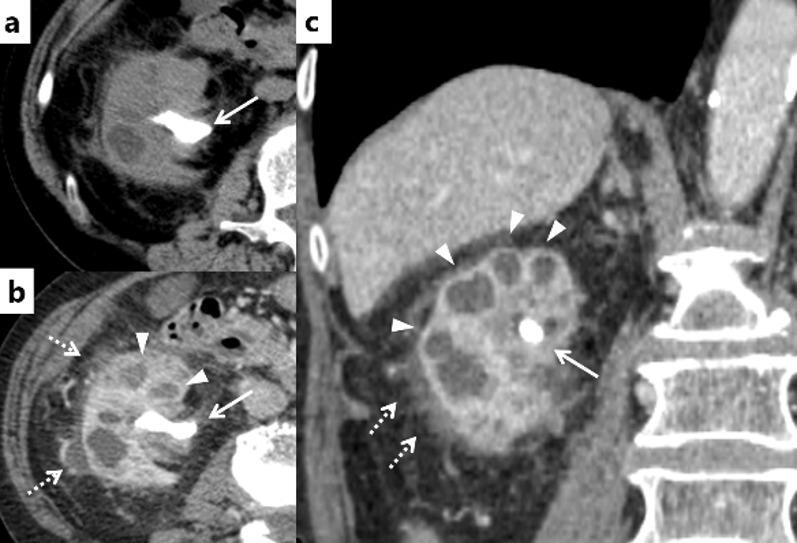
Xanthogranulomatous pyelonephritis (XGP). a Plain CT (axial image), b contrast-enhanced CT (axial image), c contrast-enhanced CT (coronal image). A woman in her 80s with type 2 DM had a right staghorn stone (a–c; arrow) and repeated chronic urinary tract infections. She presented with fever, pyuria, and elevated WBC and CRP. CT demonstrates the characteristic “bear’s paw sign,” with dilated calyces in the right kidney with contrast enhancement at the margins (b–c; arrowheads). Perinephric fat stranding is observed around the right kidney (b–c; dotted arrow). Right nephrectomy was performed, and the patient was diagnosed with XGP. Abbreviation: XGP, Xanthogranulomatous pyelonephritis; DM, diabetes mellitus; CT computed tomography; WBC, white blood cell; CRP, C-reactive protein
